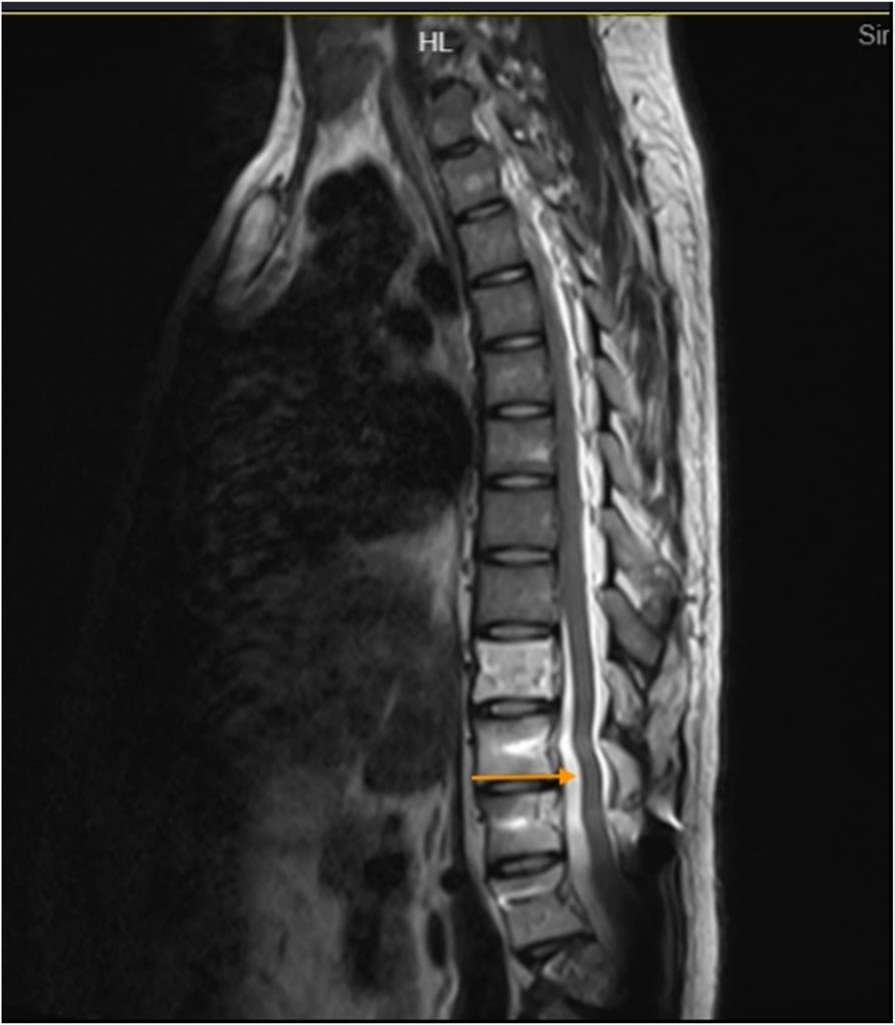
Sagittal T2-weighted MR image of the total spine showing anterior epidural fluid collections in the lower thoracic segments
